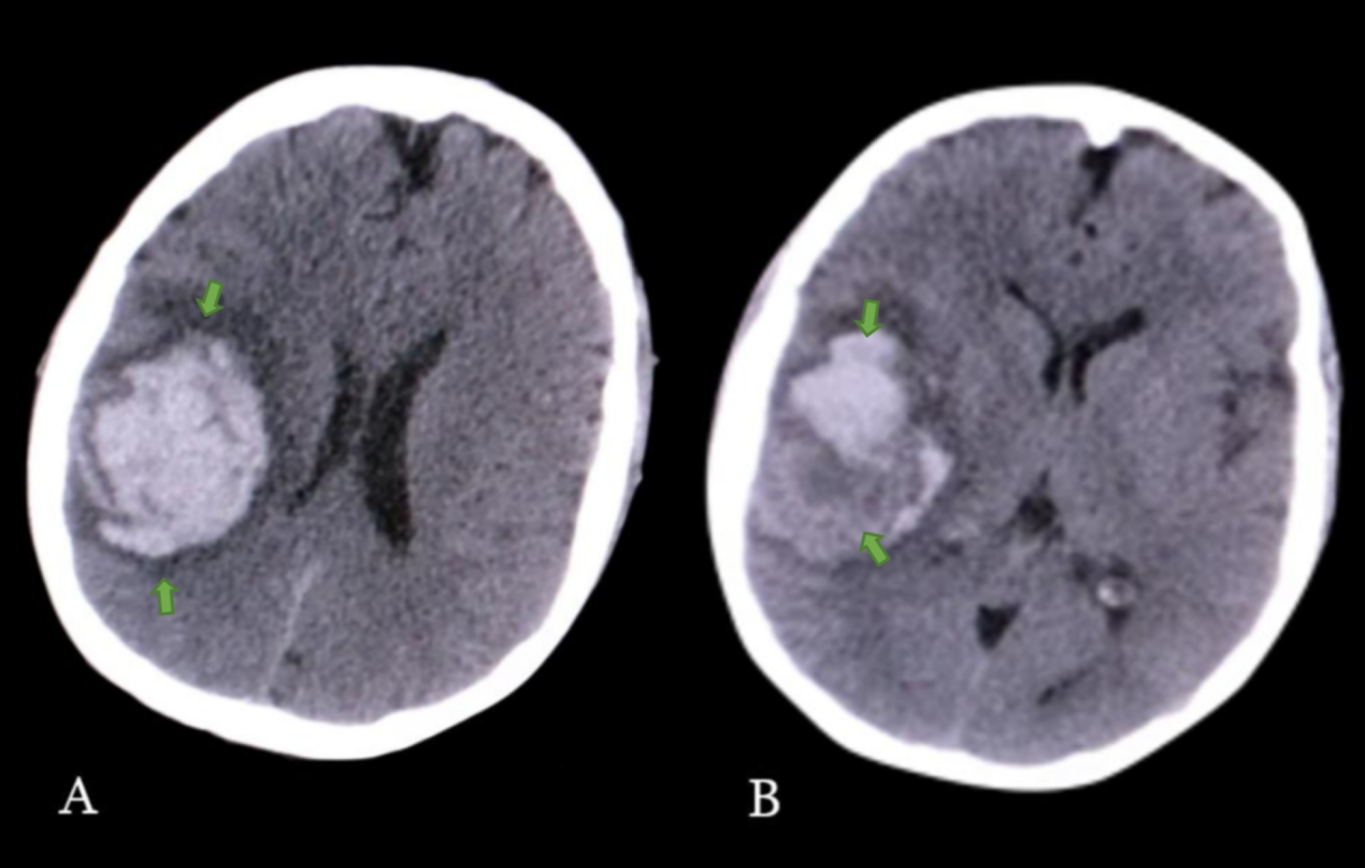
Axial section of the computed tomography (CT) scan A: Predominantly hyperdense, right frontoparietal mass-occupying lesion, suggestive of hemorrhage with midline shift; B: Heteregenous densities (hypodense and hyperdense) within the same lesion. Volume estimation of 47 mL^3^.
